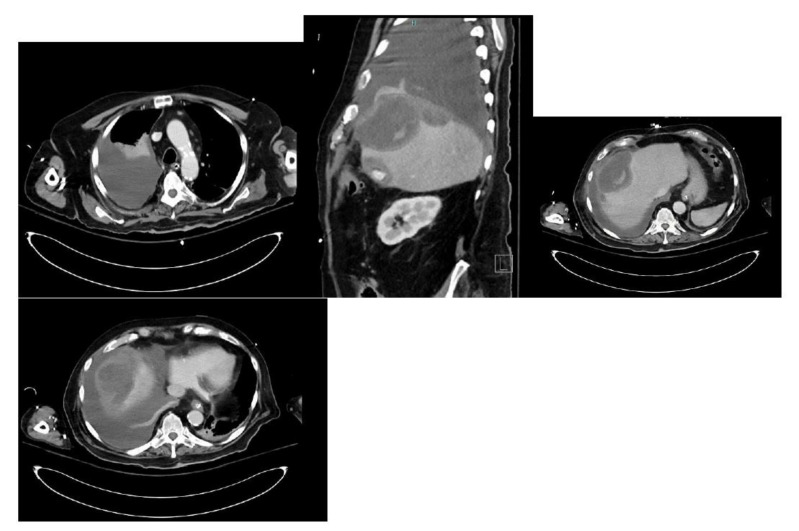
CT abdomen showing a complex low-density right hepatic lobe subcapsular lesion and a moderate right pleural effusion.
